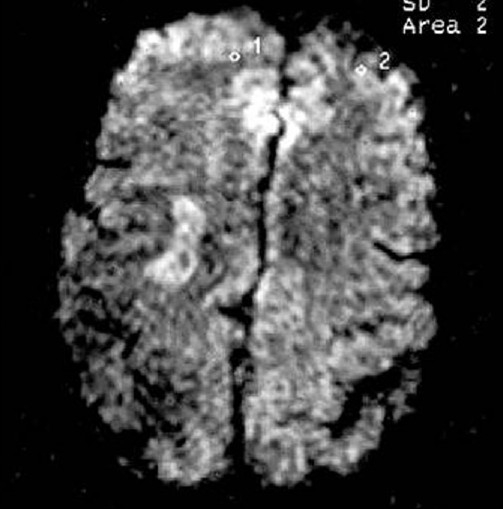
Chronic infarcts (diffusion-weighted images) suggest improved molecular diffusion of water, apparent as decreased hyperintensity in DWI at b = 1000 (second follow-up study of the same patient done 90 days after acute onset)
